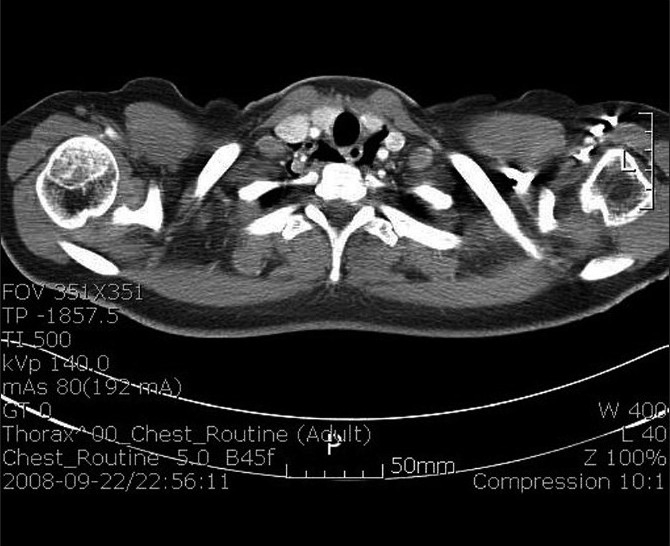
Chest CT scan of case 2 reveals pneumomediastinu
